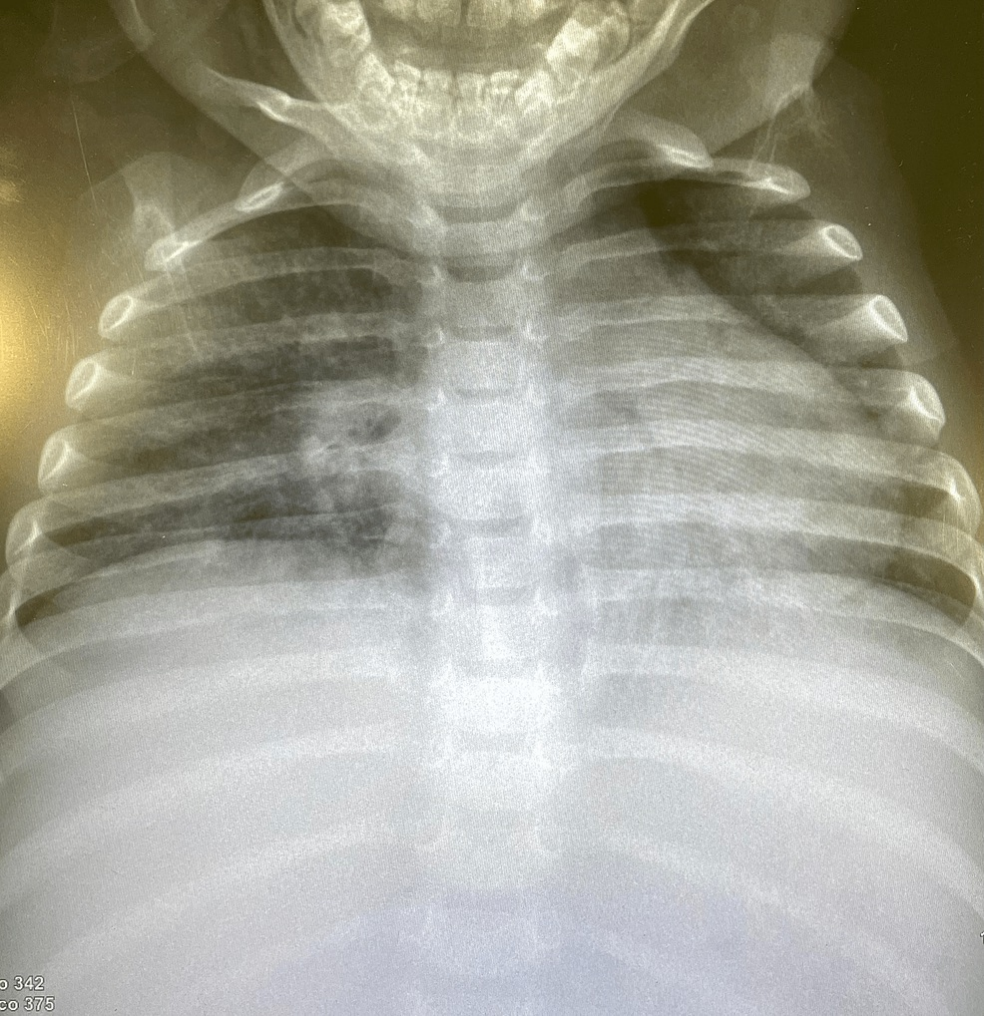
Chest X-ray image Note the increased parahilar vascularity with a parahilar infiltrate in the right lung.
